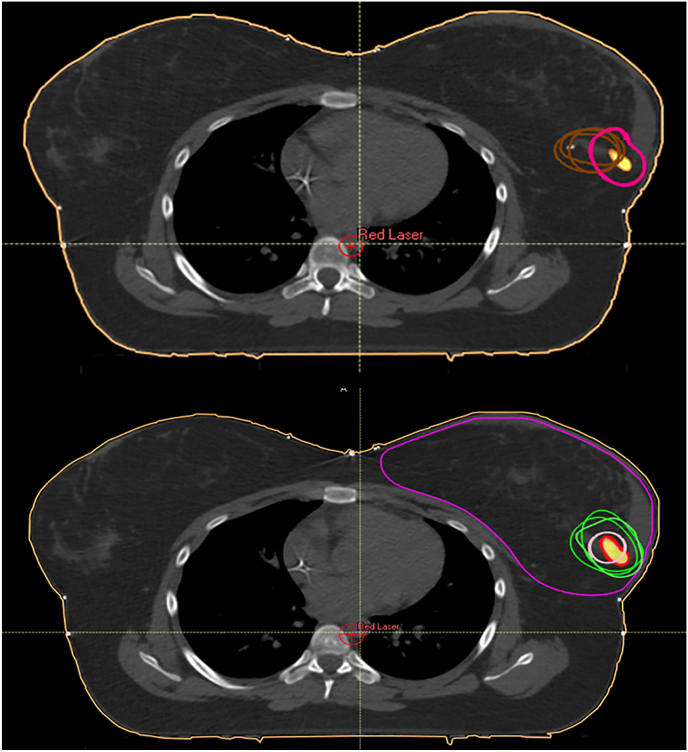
CTV_boost_ delineation strategies following deformable PET/CT–;CT fusion. Axial views after deformable registration using the CTV_breast_ as the registration volume. The PET-derived BTV (40% SUVmax) was transferred onto the planning CT for target construction. Top: CTV_CLI_ (brown) and CTV_PET_ (magenta). Bottom: CTV_COM_ (pink) and CTV_INT_ (green). (For interpretation of the references to colour in this figure legend, the reader is referred to the Web version of this article.)
